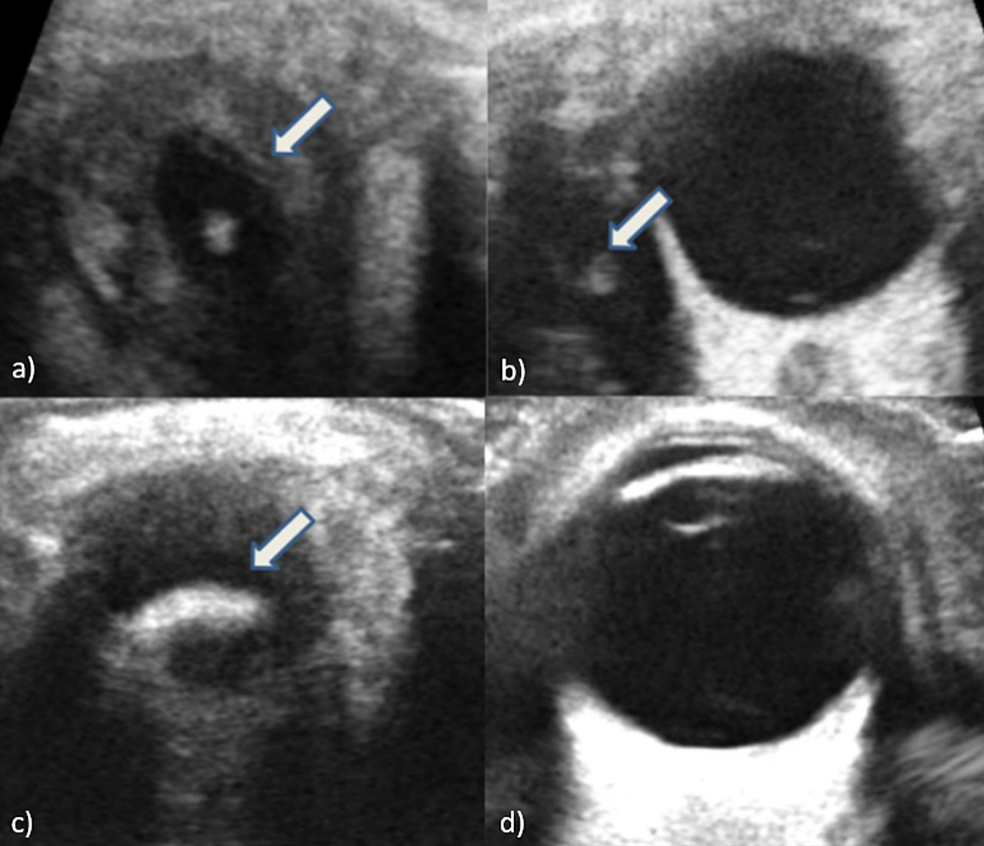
Ultrasound B scan showing a well-defined cystic lesion with an echogenic mural nodule (white arrows) in the superior rectus muscle of the left eye. (a) Transverse view just superior to the section of the eyeball showing a well-defined hypoechoic cystic lesion with a tiny echogenic mural nodule as its content within the superior rectus muscle. (b) Longitudinal view showing that the lesion is cranial to the eyeball separate from the intra-ocular compartment. (c) Transverse view of the superior rectus showing thickening and bulky muscle with peri-lesional fat stranding. (d) Transverse view of the intra-ocular compartment reveals a normal appearance with no focal lesion within.
